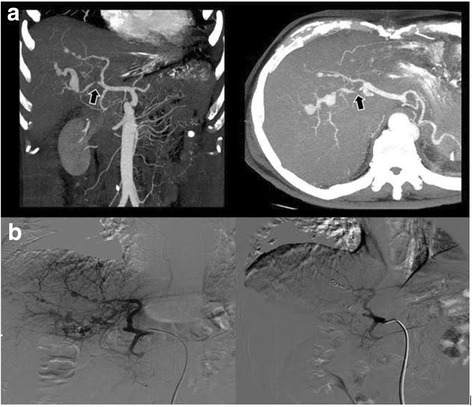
Multislice abdomen CT angiography taken on day 42 (a) showing ligation of the right hepatic artery (*arrow*) and three small MAs of the IV hepatic segment. The radiological images demonstrate residual blood flow in the right hepatic MAs. Selective angiography of the coeliac axis (b) demonstrates arterial branches from the left hepatic artery providing blood supply to the right hepatic MAs
